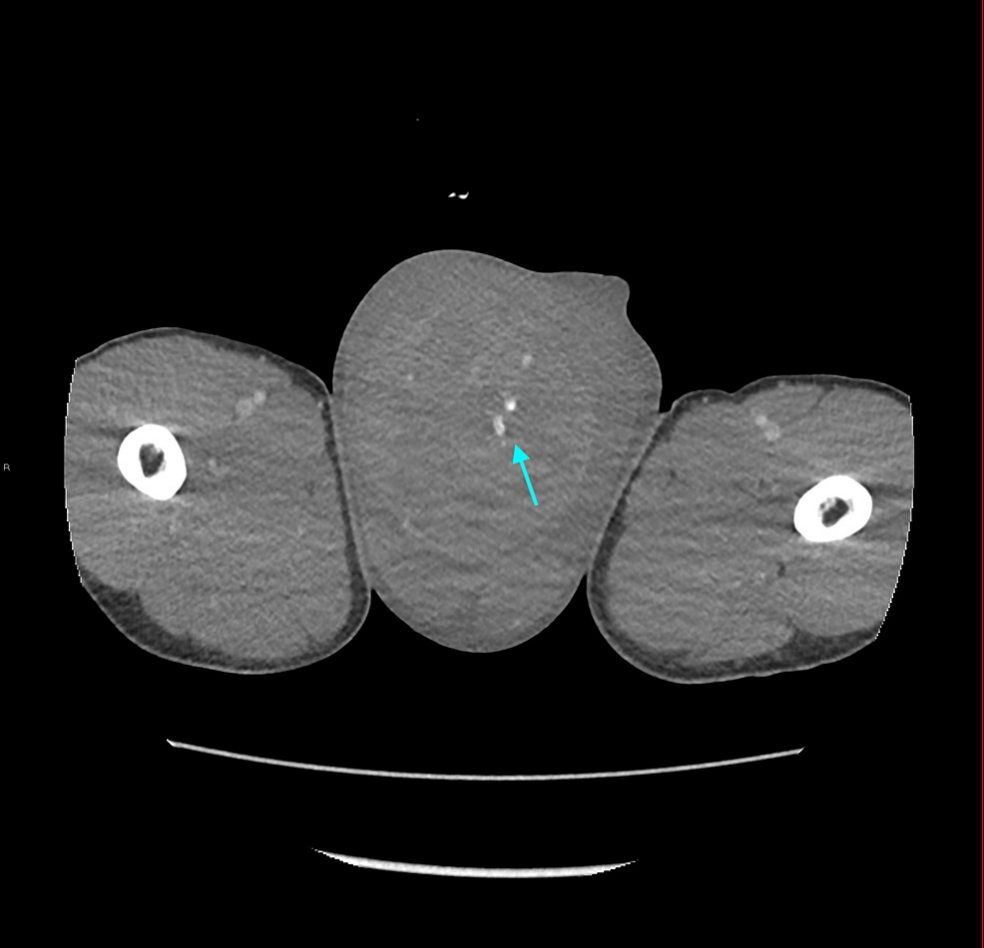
Contrast-enhanced CTA of the abdomen and pelvis demonstrating a large heterogenous collection within the right scrotum consistent with a hematoma, with evidence of active contrast extravasation (arrow) CTA: computed tomography angiography
